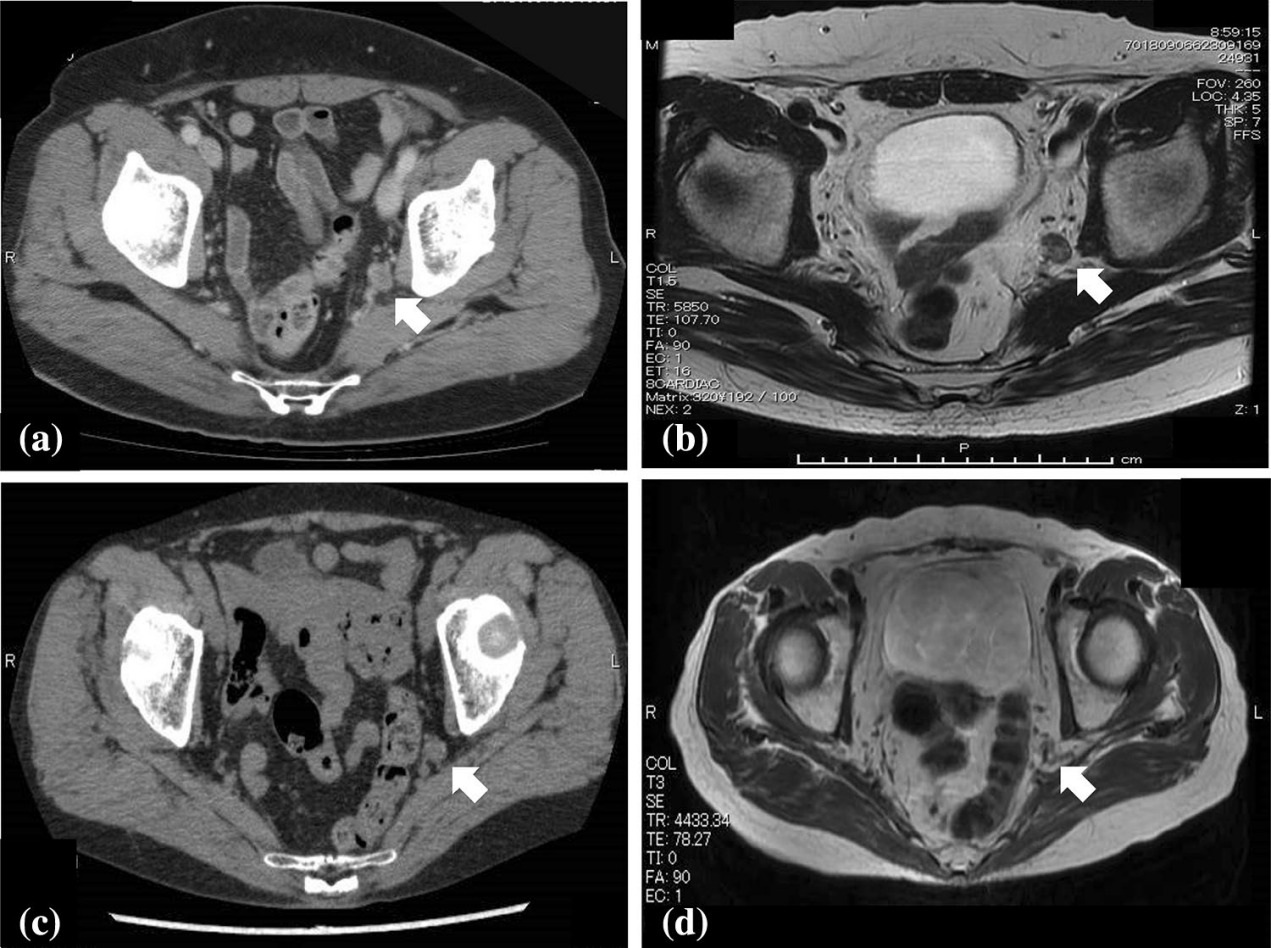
Representative abdominal computed tomography (CT) and magnetic resonance imaging (MRI) findings of patients 1 and 2. a Follow-up CT of patient 1 after primary surgery revealed left lateral pelvic lymph node recurrence. b Follow-up MRI of patient 1 after primary surgery revealed left lateral pelvic lymph node recurrence. c Follow-up CT of patient 2 after primary surgery revealed left lateral pelvic lymph node recurrence. d Follow-up MRI of patient 2 after primary surgery revealed left lateral pelvic lymph node recurrence
